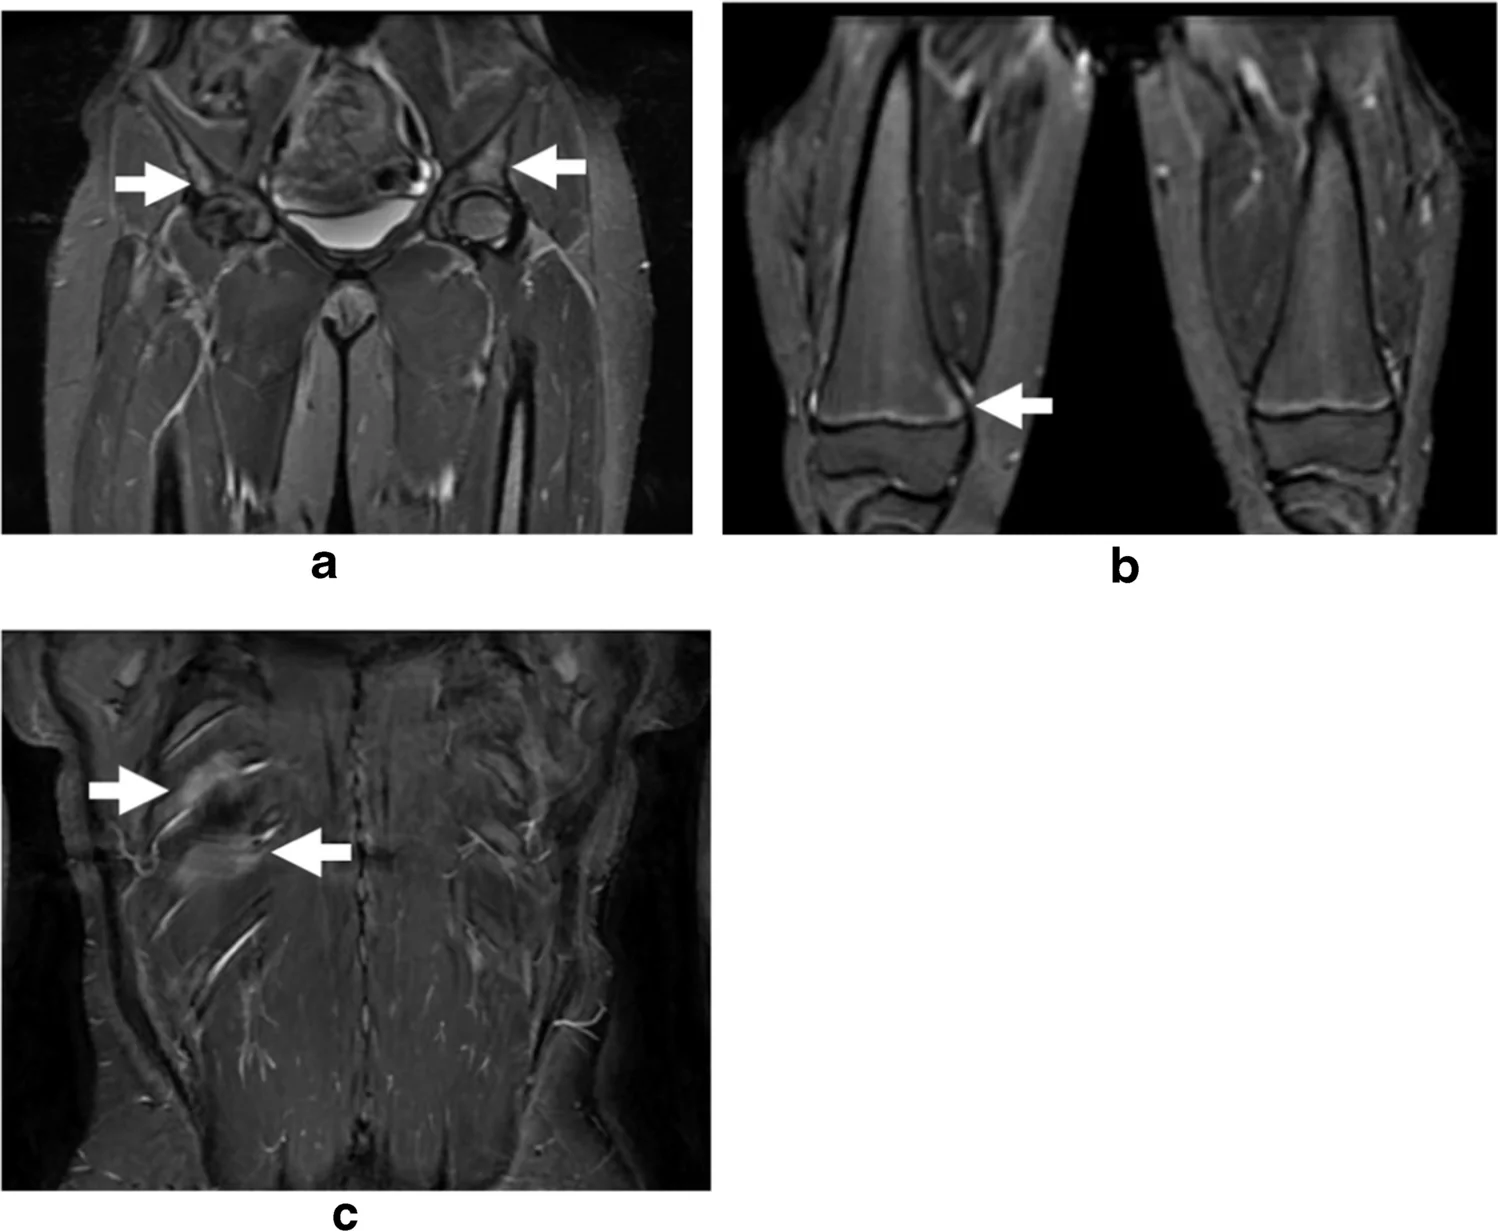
Images obtained in a 12-year-old girl with CNO involving both supra-acetabular regions, right femur, and ribs. a Coronal STIR MR image of the hips shows areas of increased signal intensity in both supra-acetabular regions (arrows). b Coronal STIR MR image of the knees shows hyperintense areas in the medial metaphysis of the distal right femur (arrow). c Coronal STIR MR image of the chest shows patchy T2 signal along the right posterior ninth and tenth ribs (arrows)
